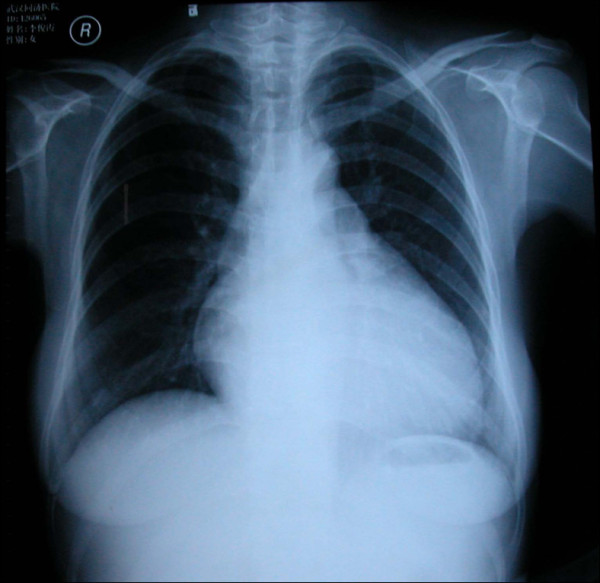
The chest X-ray showed mild increase of transverse cardiac diameter. The left cardiac border protruded left mildly.
